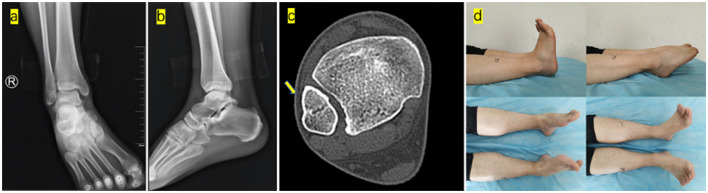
Anteroposterior (a) and lateral (b) radiographs of the right ankle at 30 months of follow-up showed normal alignment of the ankle. CT axial scans (c) show the normal syndesmosis (yellow arrow). The general images of ankle range of motion (dorsiflexion and plantarflexion) and subtalar joint range of motion showed no difference compared to the left ankle (d).
